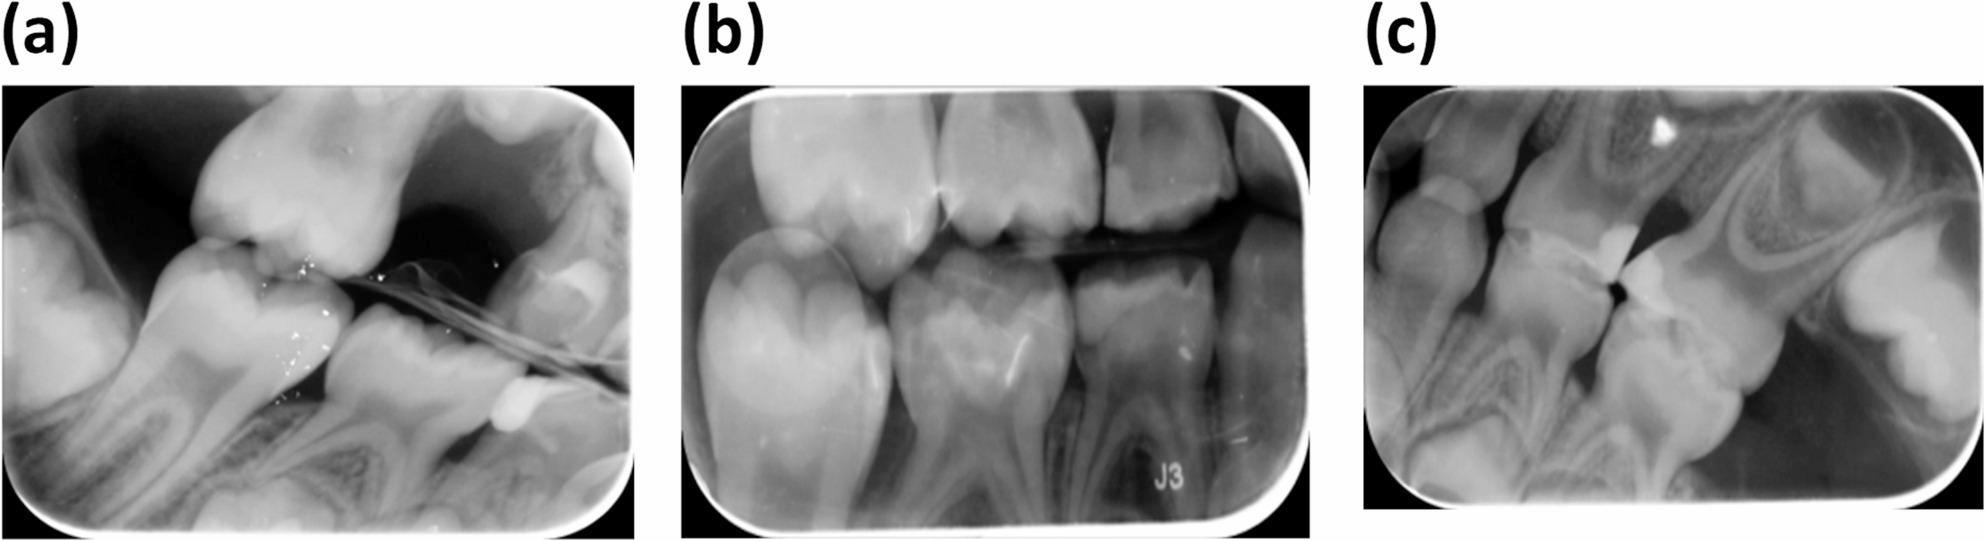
Bitewing radiographs showing postoperative assessment of the proximal overhangs of restoration (a) Positive Overhang (b) Negative Overhang (c) Absent Overhang
